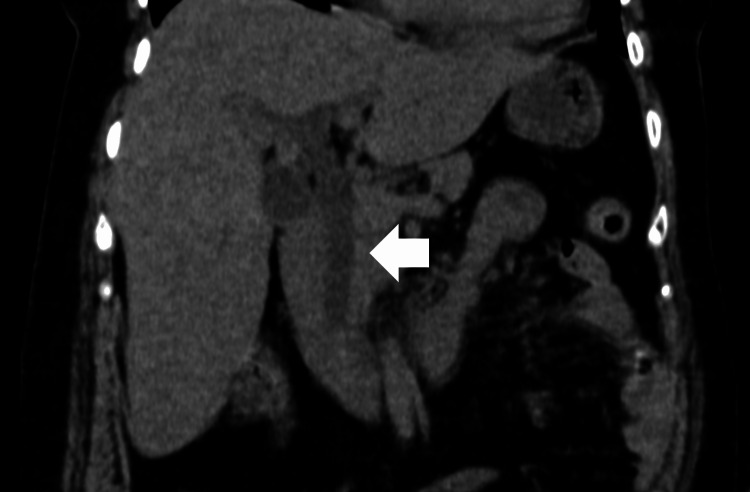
Computed tomography (CT) of the abdominal on admission demonstrates dilatation of the common bile duct (white arrow).
